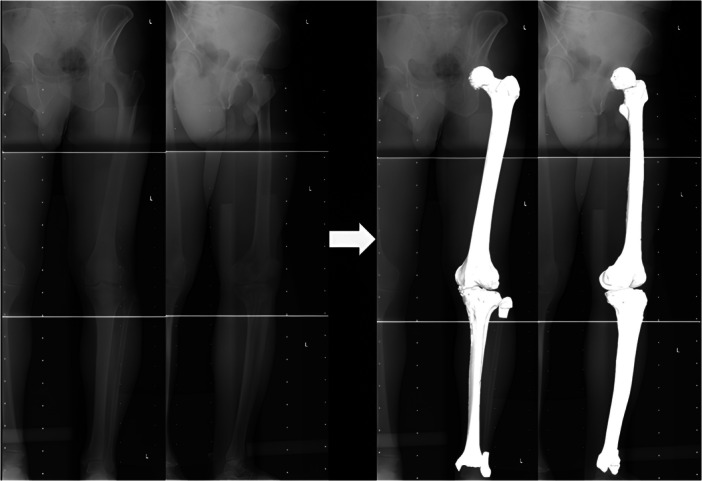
Biplanar computed radiography (CR) images of the entire lower extremities were taken in the weight bearing by standing position. The 3D digital bone models were projected onto the biplanar CR images by 3D‐to‐2D image registration technique.
